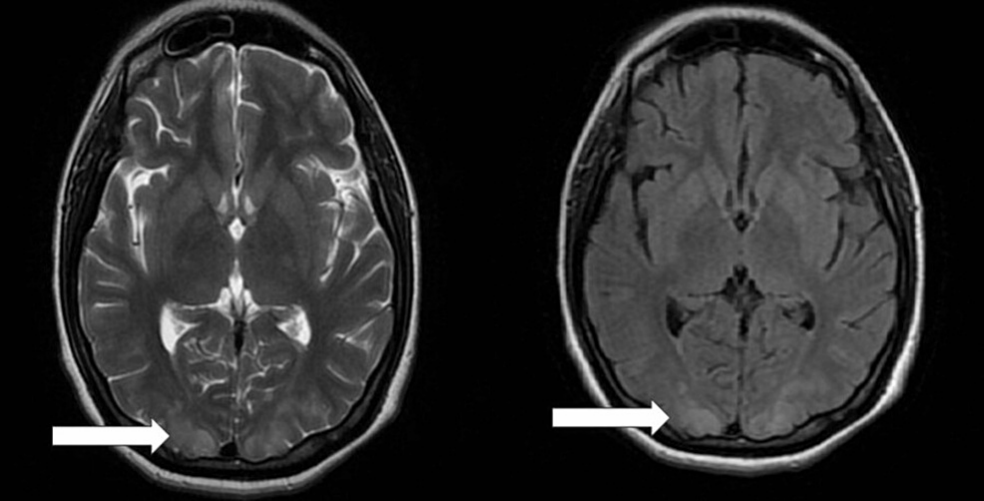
Magnetic resonance imaging of the brain showing subcortical areas of altered intensity indicated by the white arrow.
